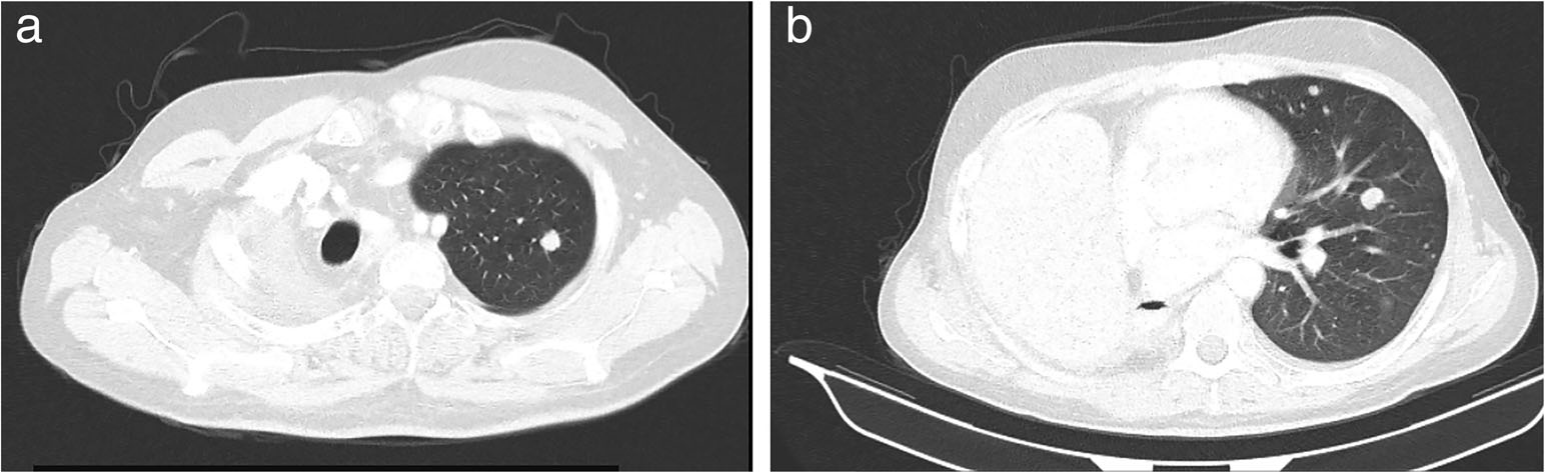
(a) Positron emission computed tomography scan at lung cancer diagnosis showing an enhanced lobulated lesion of the middle lobe of the right lung. (b) Computed tomography scan demonstrating disease progression with new pulmonary nodules in the left lung
